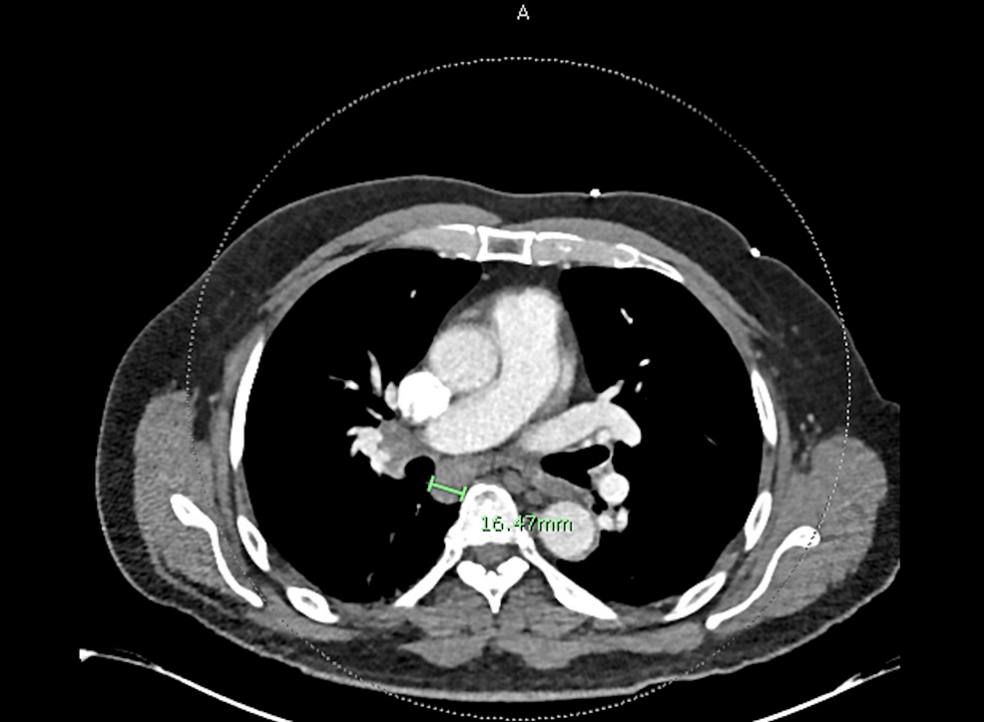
Cross section of contrast-enhanced CT scan showing 16.47 mm mediastinal lymph node
